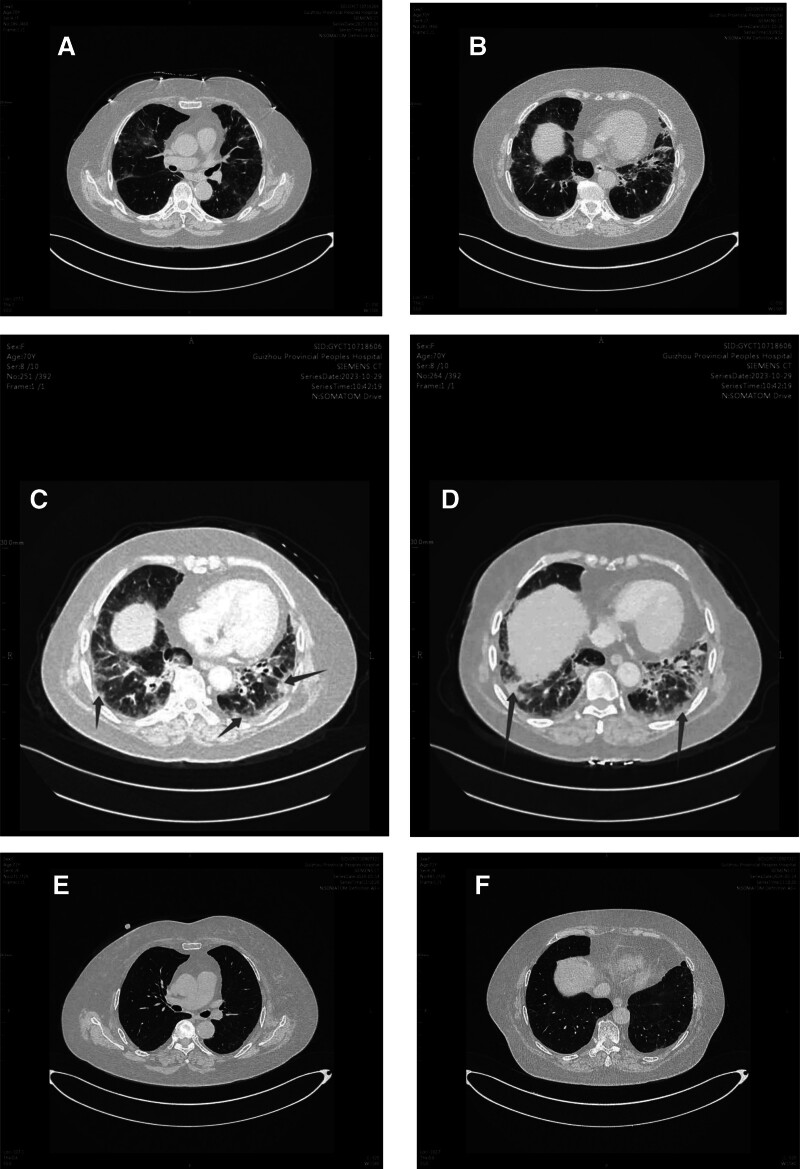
Serial chest CT images demonstrating the progression and resolution of lung inflammation during treatment. (A) Initial scan revealing multiple new inflammatory lesions in both lungs. (B) Additional view of initial scan showing extensive bilateral involvement. () Follow-up scan demonstrating multiple patchy areas of increased density in both lungs with interstitial changes, predominantly distributed subpleurally and near the pleura. (D) Corresponding view highlighting the progression of interstitial changes (arrows indicate representative lesions). (E) Final scan showing near-complete resolution of bilateral lung inflammation after treatment. (F) Corresponding view confirming significant improvement in lung condition. CT = computed tomography.
